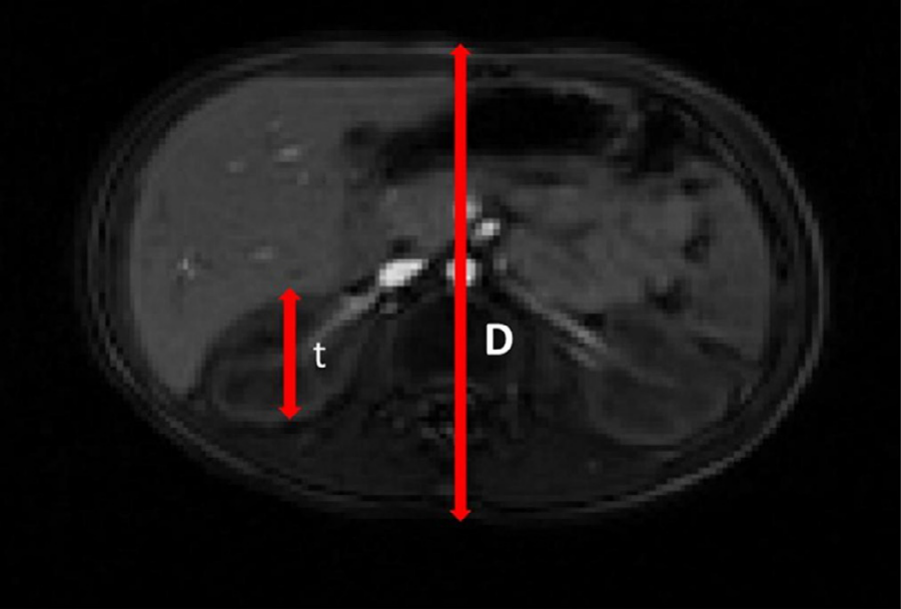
A sample of axial MRI image shown the diameter and thickness.
